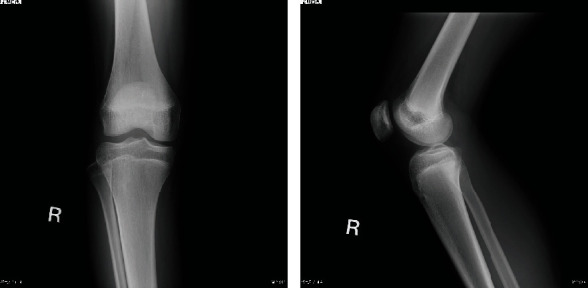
Plain radiography reveals no abnormal findings.
